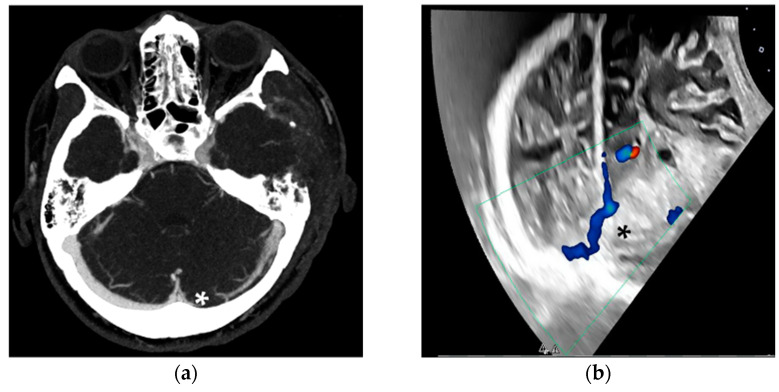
Venous CT angiography (a) and duplex ultrasound (b), both showing the occluded transverse sinus (*). The patient was later extubated, underwent cranioplasty, and recovered well. In our setting, TUS proved useful for visualizing the larger venous vessels, particularly in cases of elevated ICP, without requiring lowering the upper body for CT imaging.
